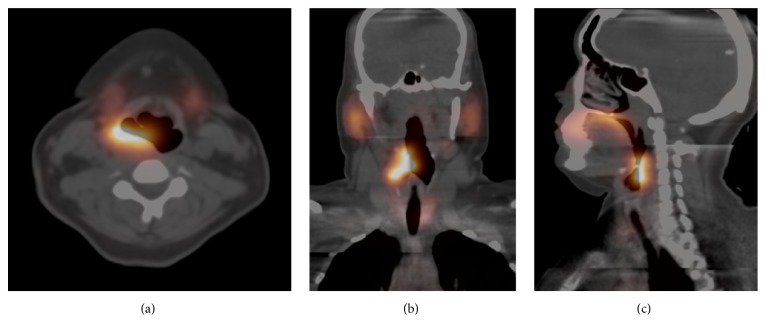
SPECT-CT images from the second ^99m^Tc-pertechnetate thyroid scan ((a) axial, (b) coronal, and (c) sagittal) localize the mass to the posterior right hypopharynx.
